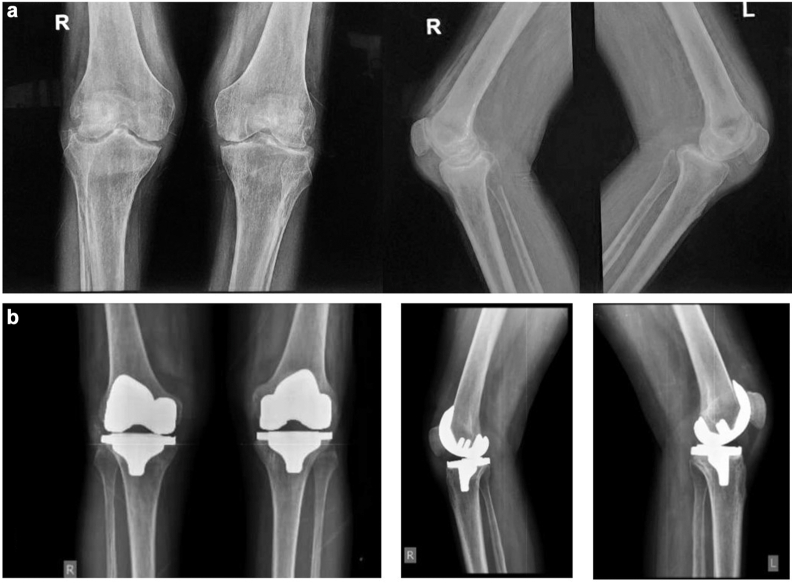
(a) Preoperative anteroposterior and lateral radiographs of both knees of case 1 demonstrating severe degenerative joint disease (DJD) with notable joint space narrowing, osteophyte formation, and significant lateral tibial plateau erosions, indicative of advanced osteoarthritic changes. (b) 24-month postoperative orthogonal radiograph of case 1 demonstrating well-fixed knee prostheses with optimal component positioning.
